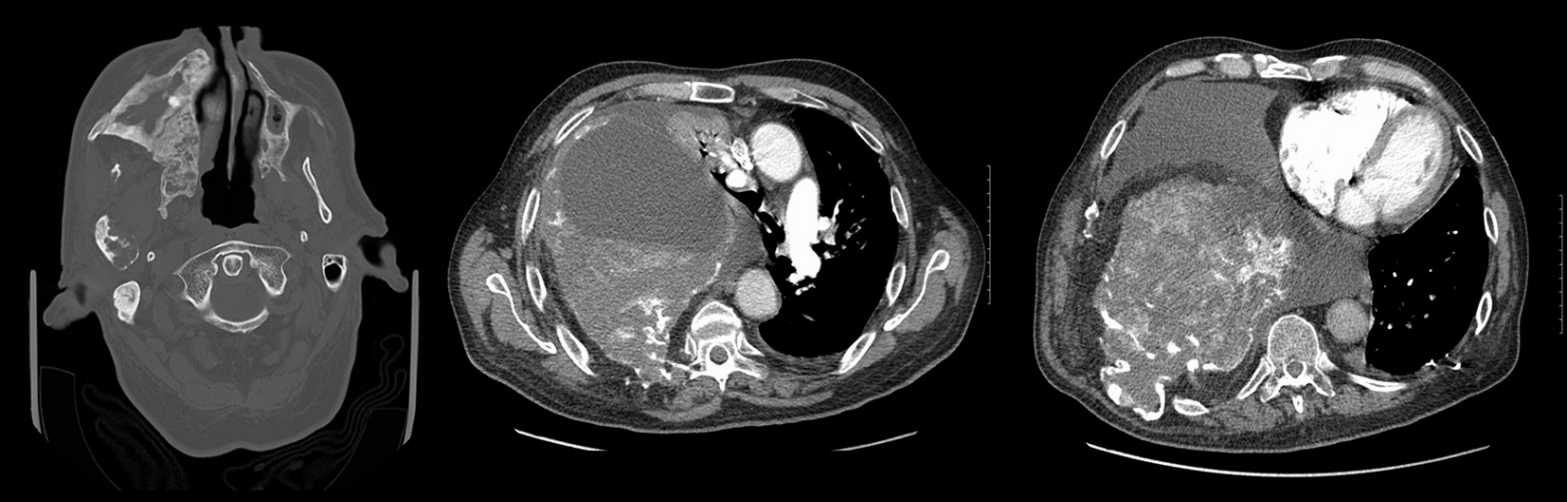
A CT scan performed 8 years later showed stability of the benign fibrous dysplasia lesions in the skull and thorax.
